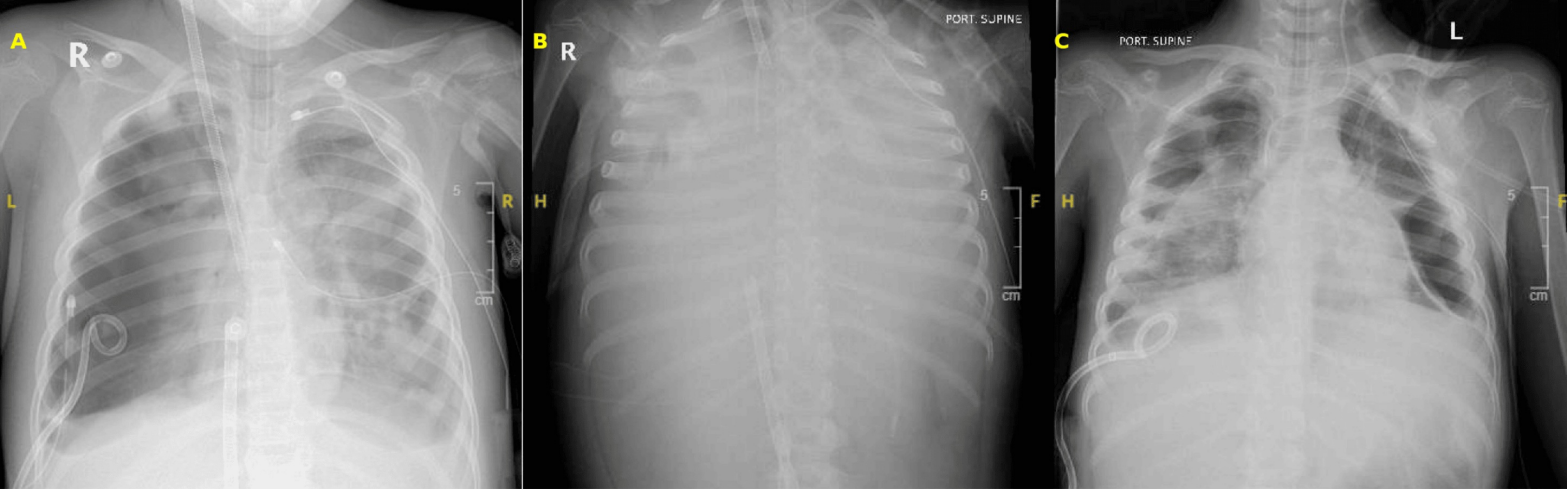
Portable supine anteroposterior chest radiographs from Case 2. (A) Day 0 on VV-ECMO: yellow “A” label marks bilateral chest tubes placed for pneumothoraces amid patchy alveolar opacities consistent with early severe pneumonia complicated by barotrauma. (B) Day 5 on VV-ECMO: yellow “B” label emphasizes “white-out” of both lungs with air–fluid levels - severe acute respiratory distress syndrome under extracorporeal support. Endotracheal tube and pleural drains are visible. (C) Day 32 (48 h post-decannulation): yellow “C” label denotes a persistent left pleural drain and residual perihilar/basal streaky opacities, compatible with a resolving bronchopleural fistula after re-expansion of both lungs.
